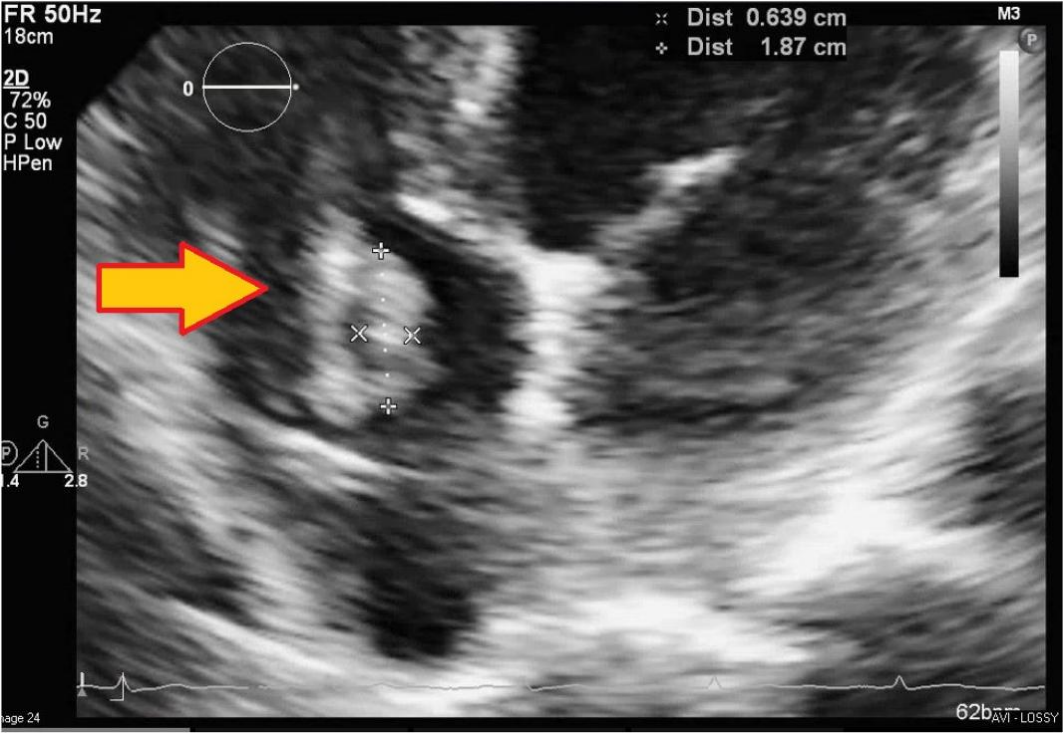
Transthoracic echocardiogram with RA mass of dimensions 2 cm × 3 cm near tricuspid valve (arrow).
